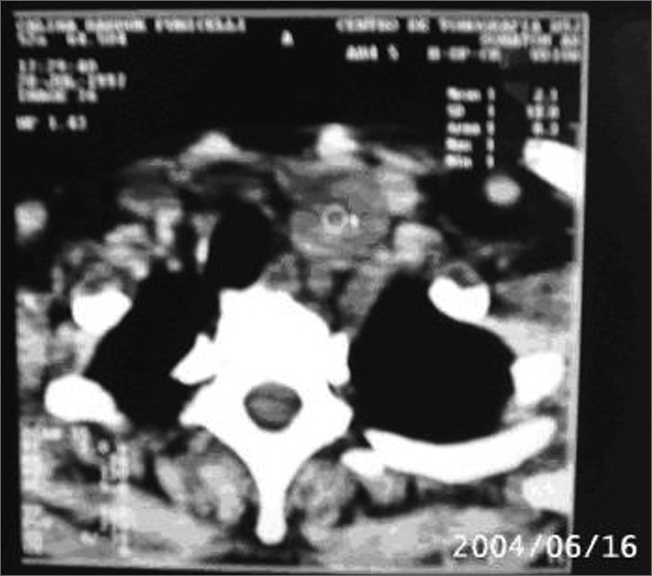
Computed tomography of the cervico-thoracic area (coronal slice) showing a tumor next to the trachea and the lower pole of the left thyroid lobe.
